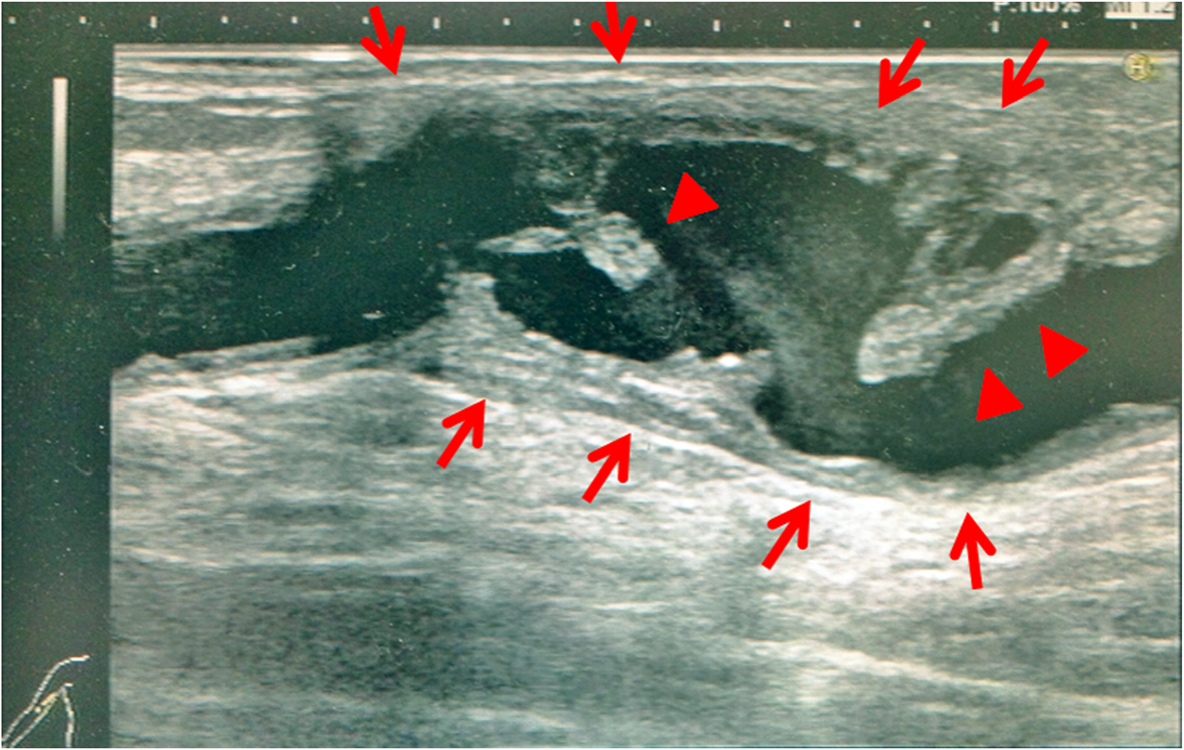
Vascular echocardiogram of the right brachial artery. An aneurysm was present on the central side of the dialysis puncture site. The aneurysm had irregular wall thickening (red arrows), and a protruding site was mobile owing to vascular pulsation (red arrowheads). This was in clear contrast to the wall thickening and arterial thrombus associated with arterial sclerosis, and it was believed to be a plaque (verruca) associated with an infection.
